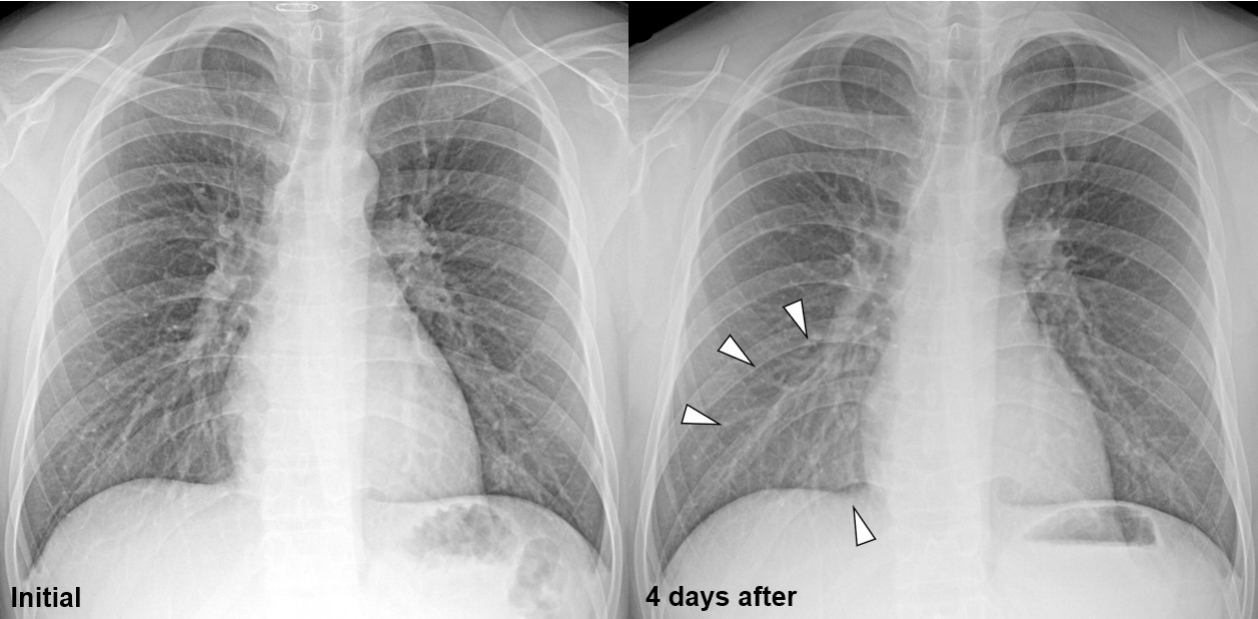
Chest radiographs (CXRs) of an initially asymptomatic patient. The CXR of a 22-year-old asymptomatic male patient. This patient had chest pain after four days, and CXR was performed. Compared with the initial CXR, increased opacity was detected in the right lower lung zone (arrowheads), suggestive of pneumonia. However, the patient’s symptoms were not aggravated and the medical staff decided to observe this patient in CTC, rather than transfer him to a hospital. The opacity had disappeared at the time of discharge.
